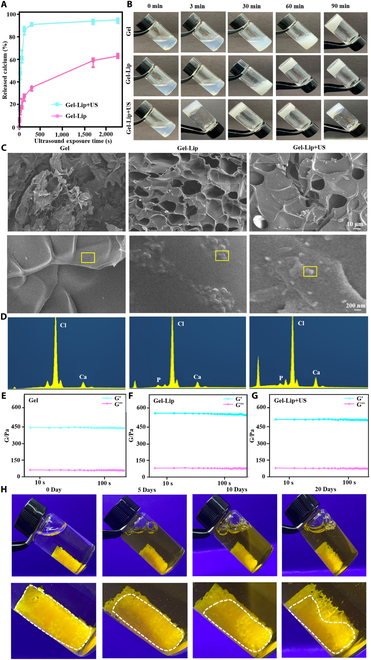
Characterization of genetic engineered injectable hydrogels. (A) Release of calcium ions by liposomes triggered by ultrasound. (B) Cross-linking time and cross-linking status of each hydrogel. (C) SEM images of hydrogels and distribution of surface liposomes. (D) EDS carried out elemental analysis of hydrogel surface conditions. (E to G) Rheological analysis results of Gel, Gel-Lip, and Gel-Lip+US hydrogel. (H). Immersion in a balanced solution after the hydrogel has formed in the artificial bone. The white line is the outline of the hydrogel in the artificial bone.
